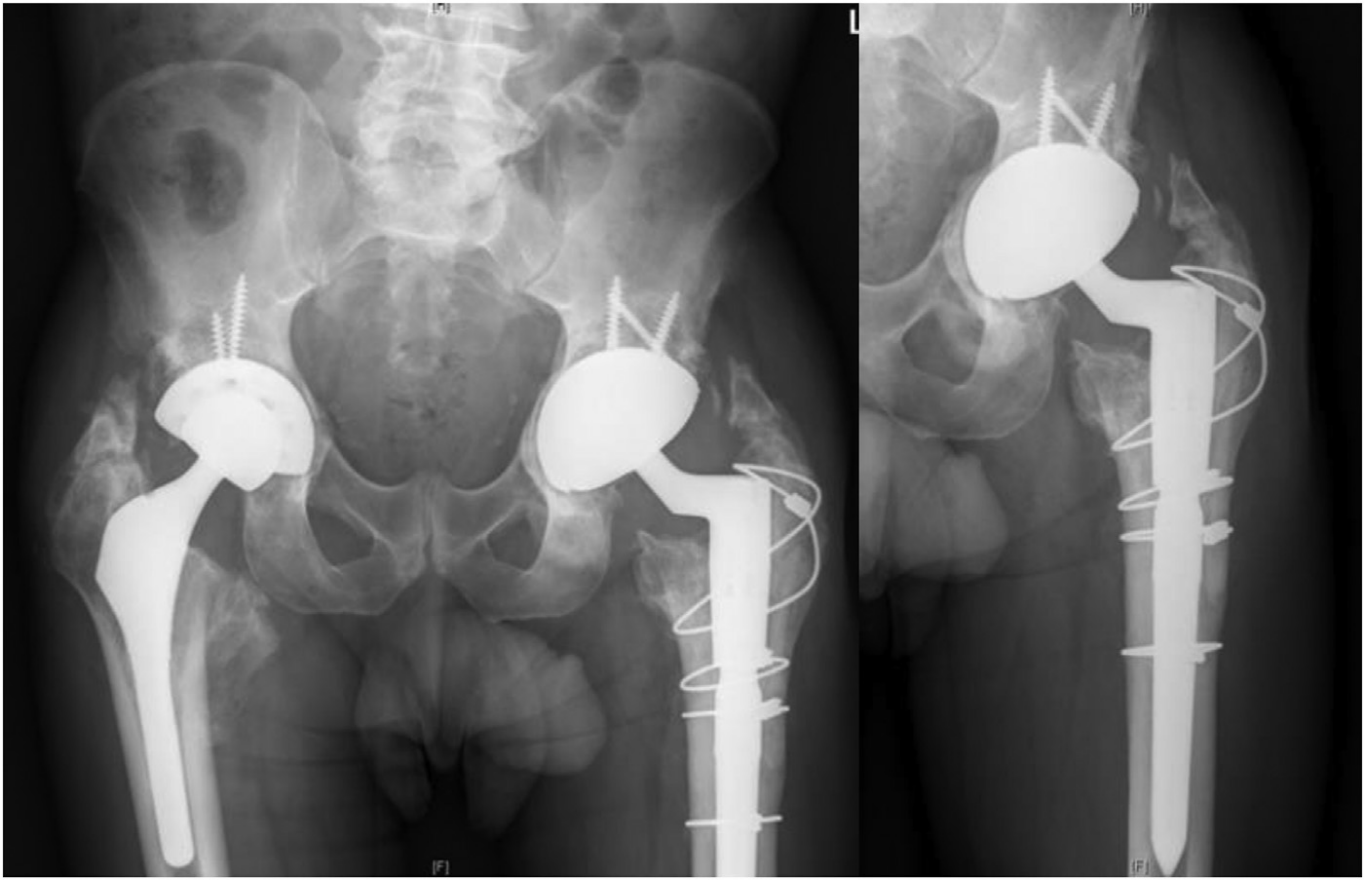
Postoperative radiographs 1 year from left and 6 months from right revision hip arthroplasty.
